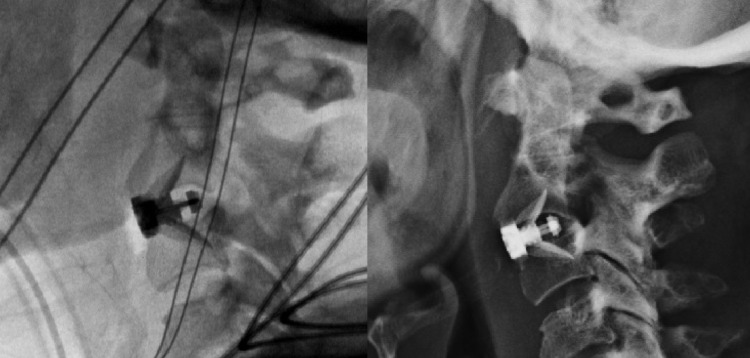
Intraoperative fluoroscopy (left) following the placement of a zero-profile, stand-alone implant at C2/3. The device position was noted to be stable on a six-week postoperative lateral radiograph.
